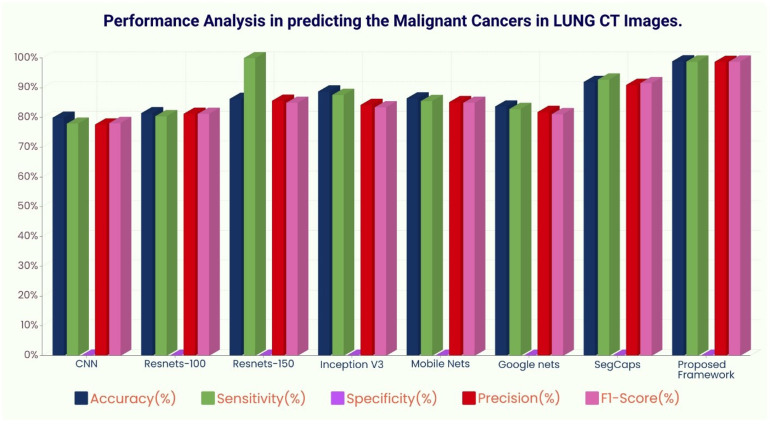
Performance analysis in predicting malignant cancer in lung CT images.
